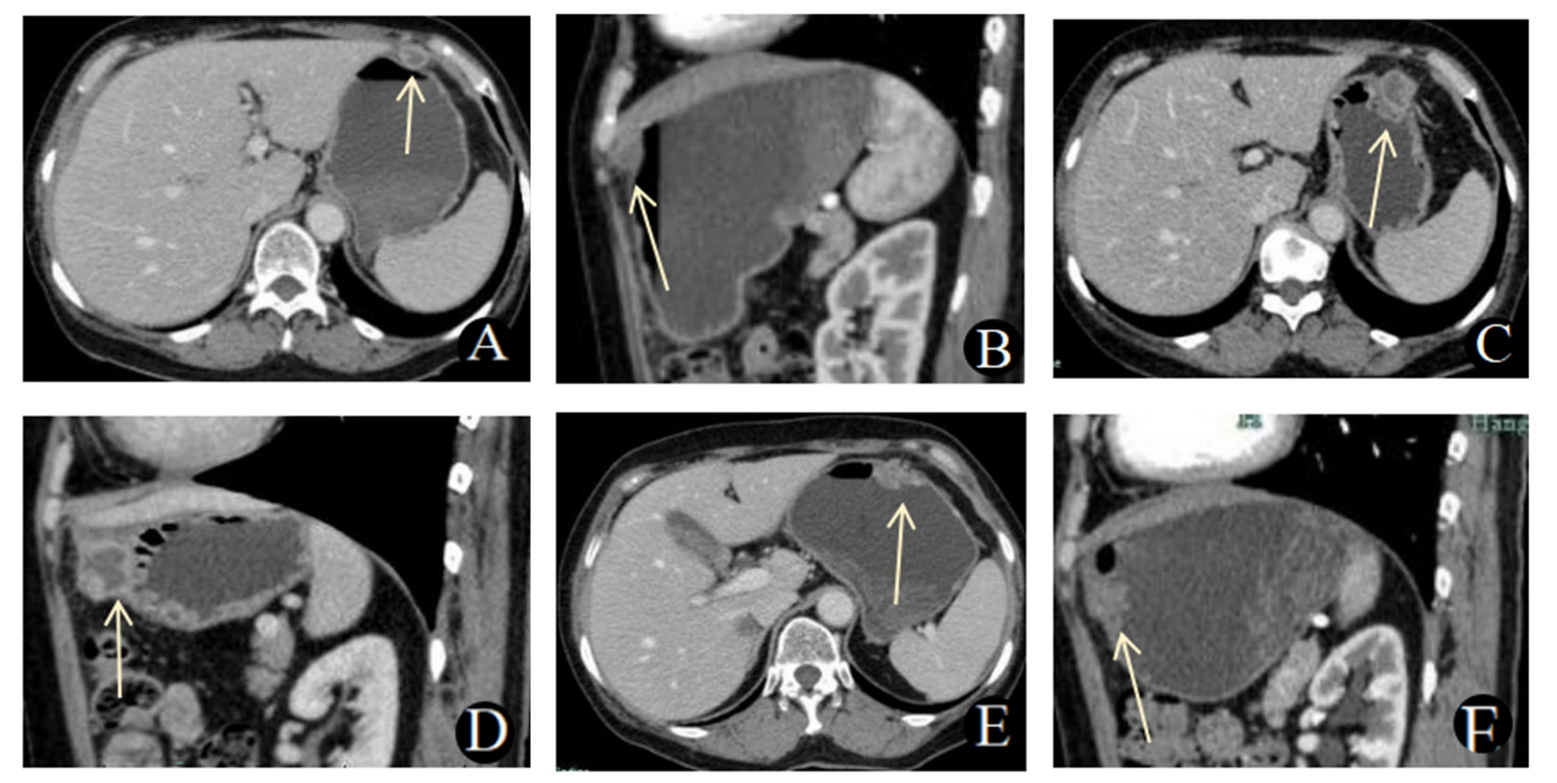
(A,B) Contrast-enhanced abdominal CT scan on August 17, 2023. (A) Axial CT scan and (B) sagittal reconstruction showed a ring-enhancing nodule adjacent to the anterior gastric wall, with the gastric wall remaining intact. (C-D) Contrast-enhanced abdominal CT scan on February 27, 2024. (C) Axial CT scan and (D) sagittal reconstruction revealed that the irregular nodule near the anterior gastric wall had grown larger and involved the gastric wall. (E,F) Contrast-enhanced abdominal CT scan on June 27, 2024. (E) Axial CT scan and (F) sagittal reconstruction showed that the gastric wall lesion had further enlarged and penetrated the gastric wall.
